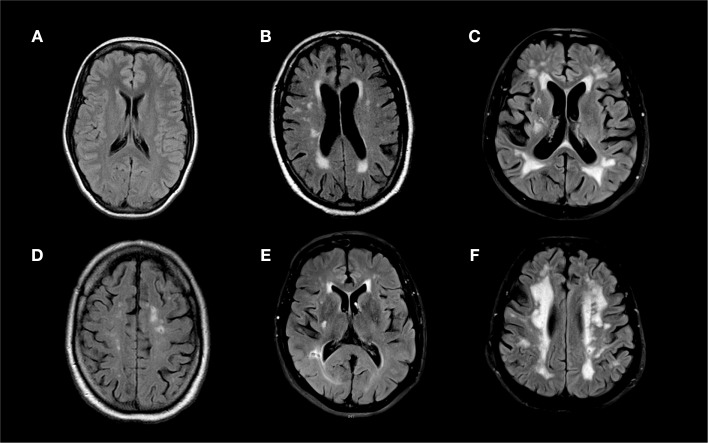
Fluid attenuated inversion recovery (FLAIR) MRI sequences of (A) a 21 year old patient with an overall Wahlund score of 0, (B) a 51 year old patient with an overall Wahlund score of 8, (C) a 69 year old patient with a Wahlund score of 20, (D) a 75 year old man with an overall Wahlund score of 12 and a 4 mm cavitating lacune in the left parietal lobe, (E) a 63 year old man with an overall Wahlund score of 5 and a 5.5 mm cavitating lacune in the right occipito-temporal region, (F) a 70 year old man with an overall Wahlund score of 20 and an 4 mm cavitating lacune in the left pareito-temporal region.
